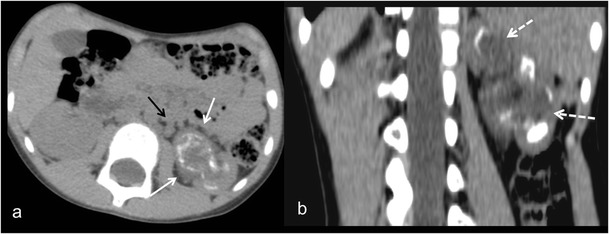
Atypical diffuse XPN. a Transverse and b coronal reconstructions CT show an atrophied kidney with multiple lithiasis, dystrophic calcifications in low attenuation areas of parenchyma destruction (*discontinuous arrows*), perirenal extension (*white arrows*) and retroperitoneal adenopathies (*black arrows*)
